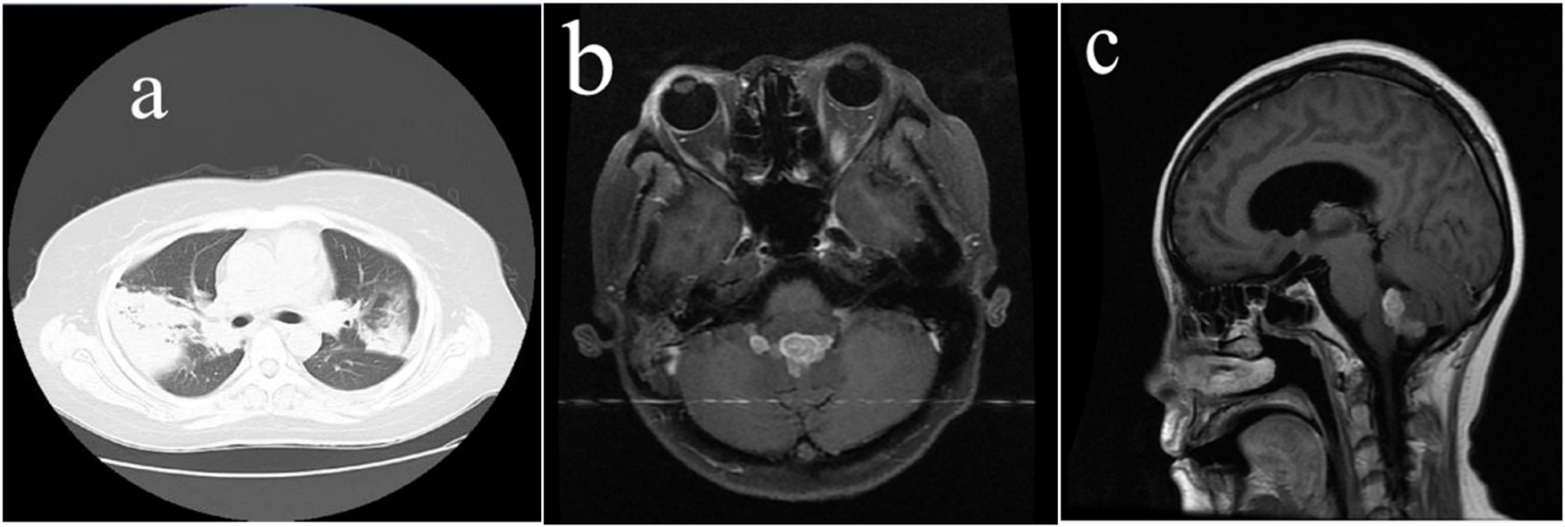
(a) Chest CT showing large patches and a patchy increased density in both lungs, and multiple bronchial stenoses in both lungs. (b and c) Head MRI showing irregular masses in the fourth ventricle, with a larger cross-section of approximately 21 mm × 24 mm, spreading along the bilateral lateral foramen of the fourth ventricle, and the sinus bone and surrounding pia mater were intact.
